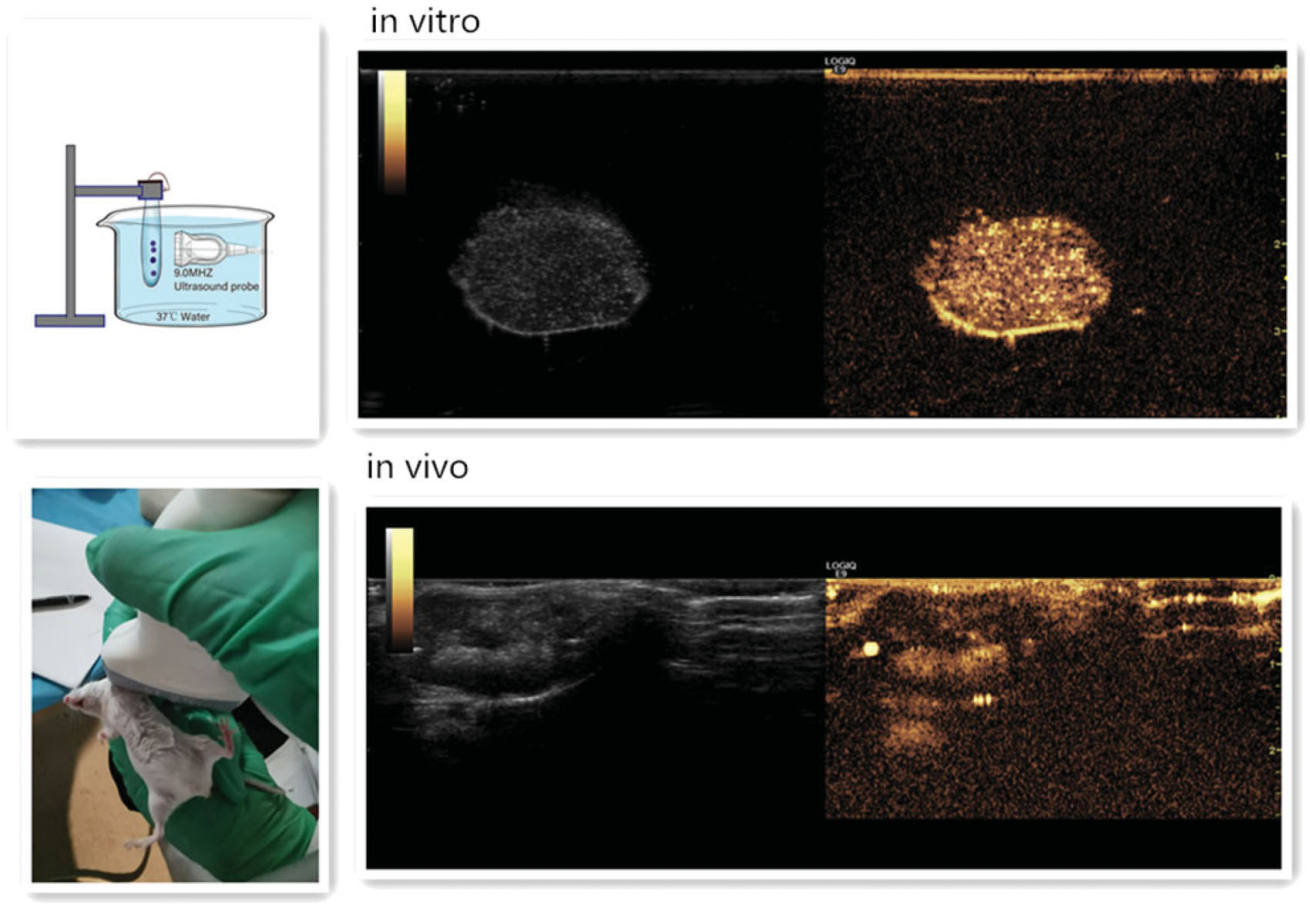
Ultrasound enhancement images of DOX-BCNDs *in vitro* and *in vivo*.
